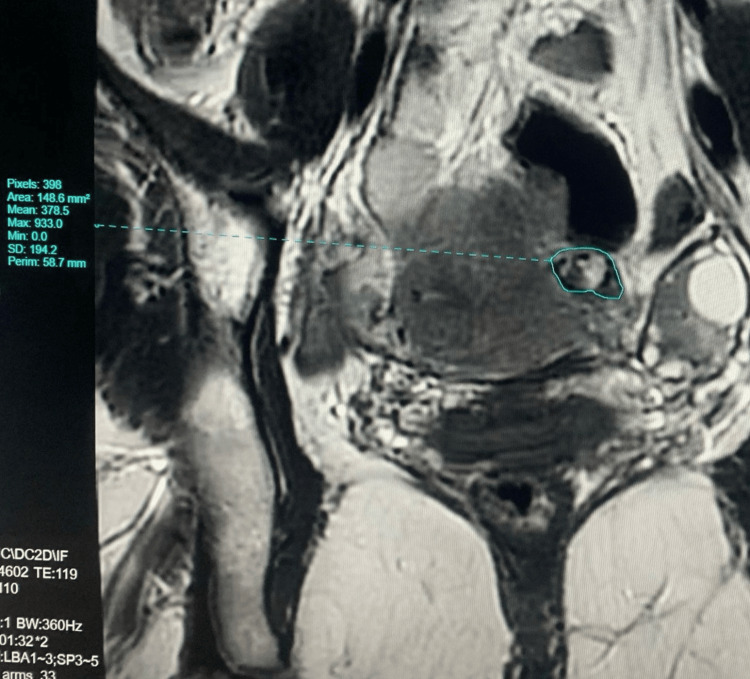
MRI image of left interstitial ectopic pregnancy: 17x12 mm-sized well-defined gestational sac is seen eccentrically located near the left uterine cornu
